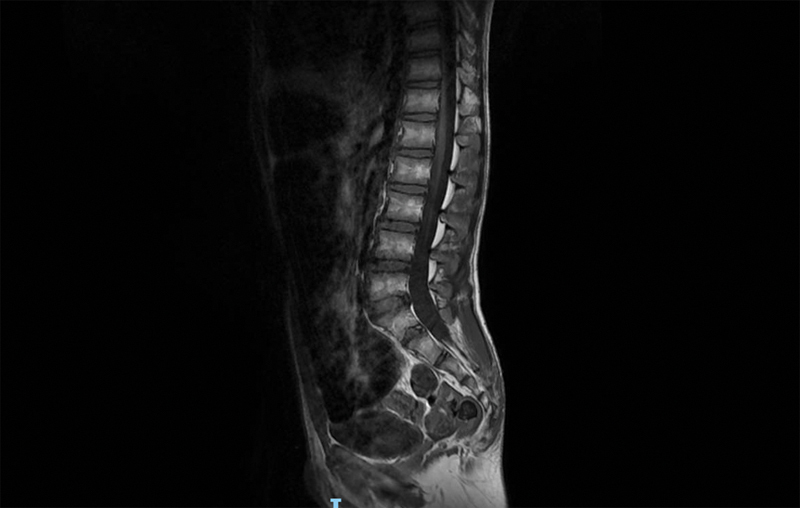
Spine magnetic resonance imaging showing S5 posterior arch cleft and distal sacral lipomatosis.
